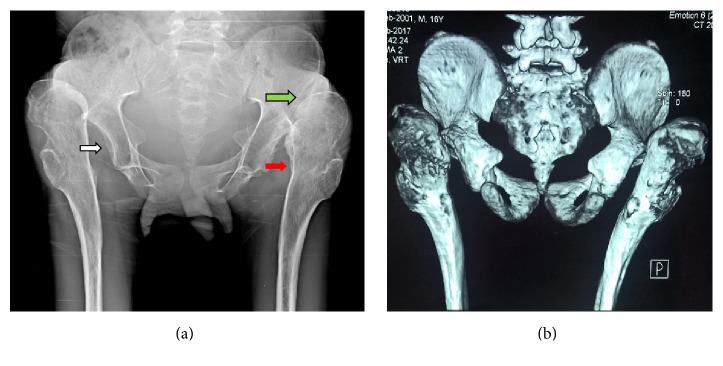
Anteroposterior radiograph of pelvis with both hip joints (a) showed widening of pubic symphysis, bilateral shallow acetabulum (white arrow), abnormal contour of bilateral femoral head with increased neck shaft angle (coxa valga deformity) (red arrow), enlarged and elongated bilateral greater trochanter, superolateral migration of bilateral femoral head overlying adjacent to iliac bones (green arrow), and slender proximal femoral shafts. CT of pelvis (b) confirming pubic diastasis, bilateral shallow acetabulum, bilateral coxa valga deformity, bilateral hip dislocation, and pseudoarticulation of the left femoral head with iliac bone.
